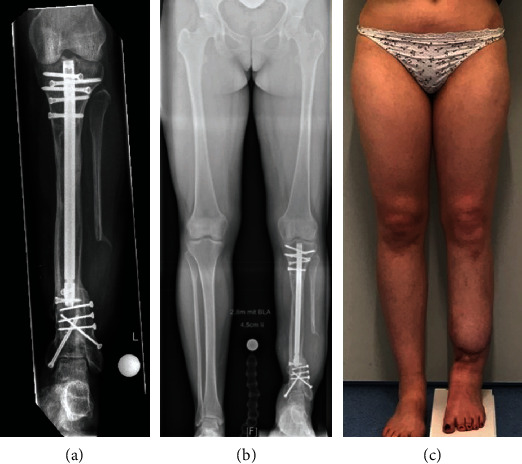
Patient 1. (a) Radiograph showing complete consolidation of the entire tibia including the former callotasis site and the distal docking site with autologous bone grafting using a part of the distal fibular diaphysis as well as trauma nail and multiple screw osteosynthesis. (b) Bilateral long-standing radiograph showing correct alignment but persistent shortening of the reconstructed leg. (c) Clinical presentation showing persistent leg length discrepancy.
